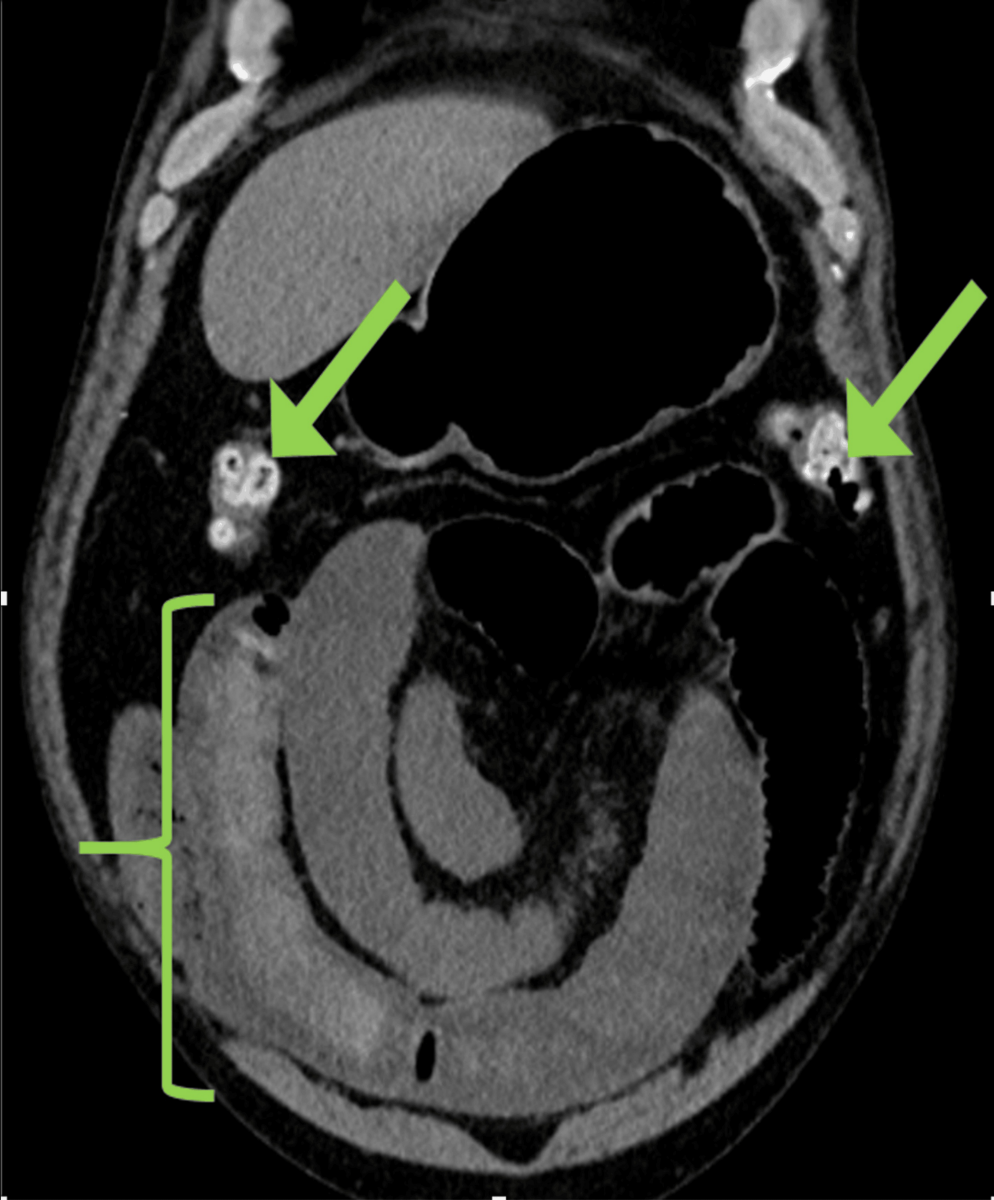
CT coronal imaging, demonstrating colonic scybala associated with opioid-induced chronic constipation (arrows) and residual distal ileal intraluminal barium after modified barium swallow (bracket)
